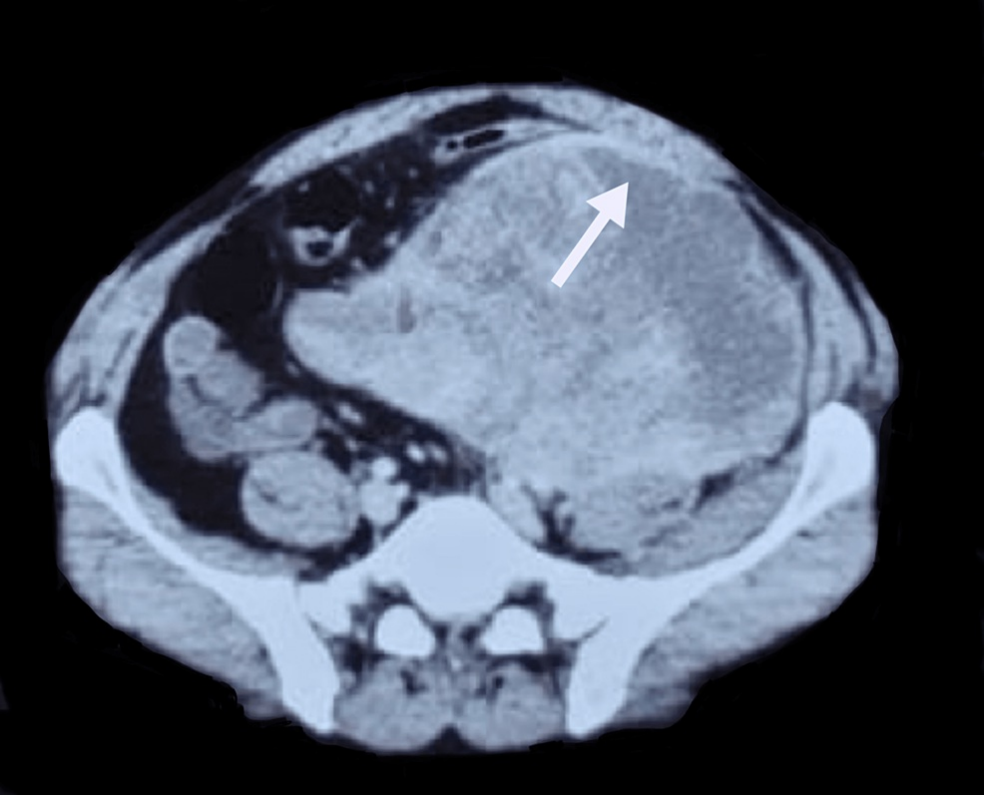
CT scan showing the tumor mass originating from iliopsoas muscle with patchy contrast enhancement of the mass.
